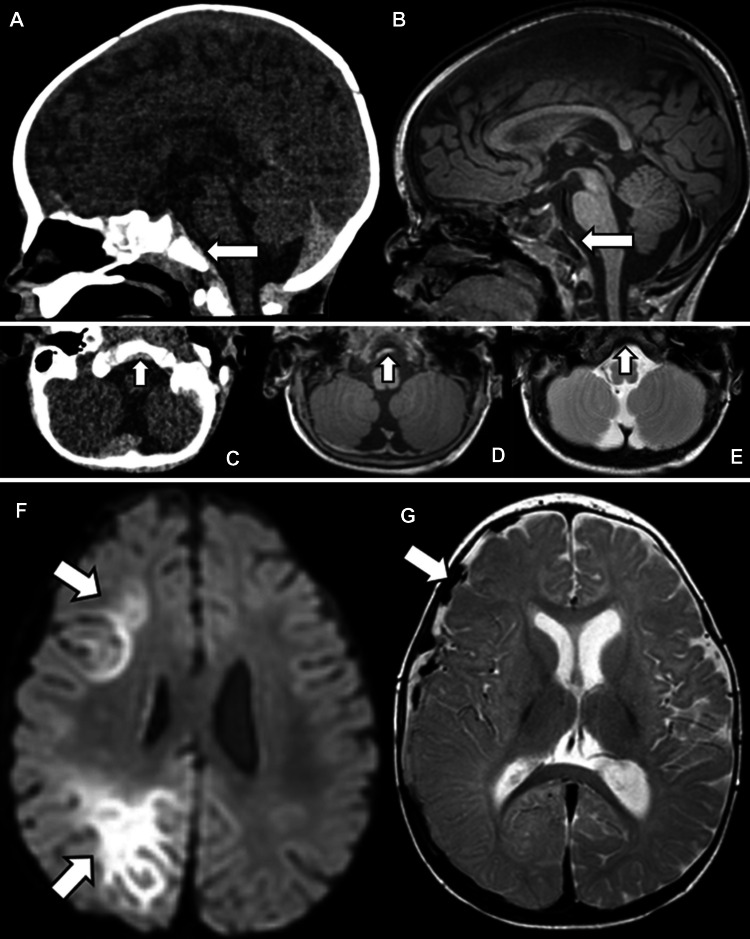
A 6-month-old male with abusive head trauma and retroclival hematoma on CT and MRI performed 3 days later. Sagittal CT (A) and T1-weighted MRI (B) demonstrate posterior fossa SDH, including hyperattenuating, T1-hyperintense retroclival hematoma (*arrows*). Axial CT (C) and T1-weighted (D) and T2-weighted (E) MR images of the posterior fossa show the hyperattenuating, T1-hyperintense, T2-hypointense hemorrhage (*arrows*). Axial diffusion-weighted (F) and T2-weighted (G) MRI of the brain show hypoxic injury (*arrows* in F) and mixed signal intensity SDH with injured bridging veins (*arrow* in G). CT and MRI are often complementary in the detection of small retroclival hemorrhages, and evaluation of both modalities can increase diagnostic confidence
